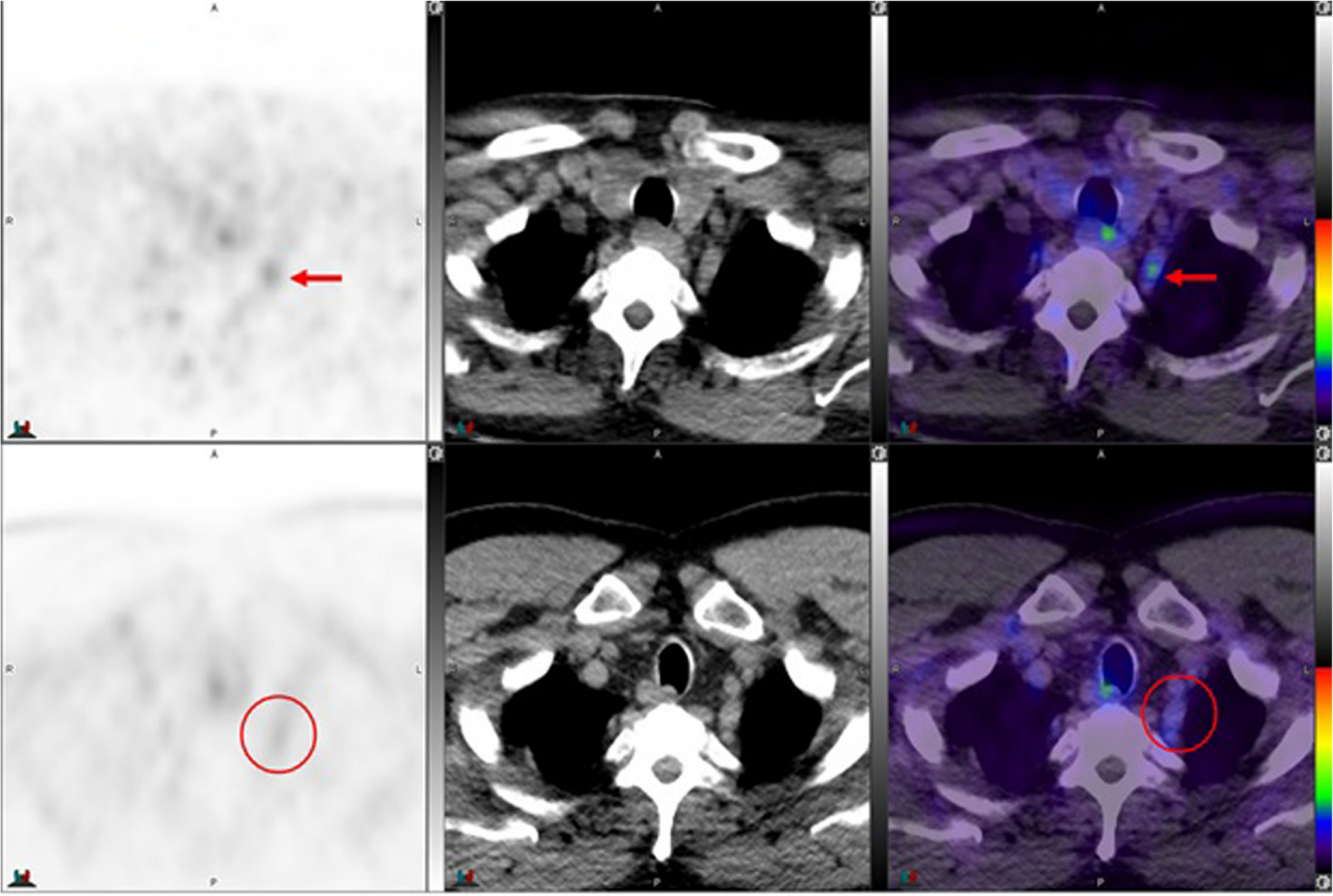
Top panel ^68^Ga-PSMA-11: axial PET, axial CT and axial fused PET/CT. Lower panel ^18^F –DCFPyL: axial PET, axial CT and axial used PET/CT. The color intensity has been slightly adjusted to highlight the uptake in the ganglia (arrows in the top panel and circles in the lower panel). Left stellate ganglion uptake with ^68^Ga-PSMA-11 only (SUVmax = 1.8)
